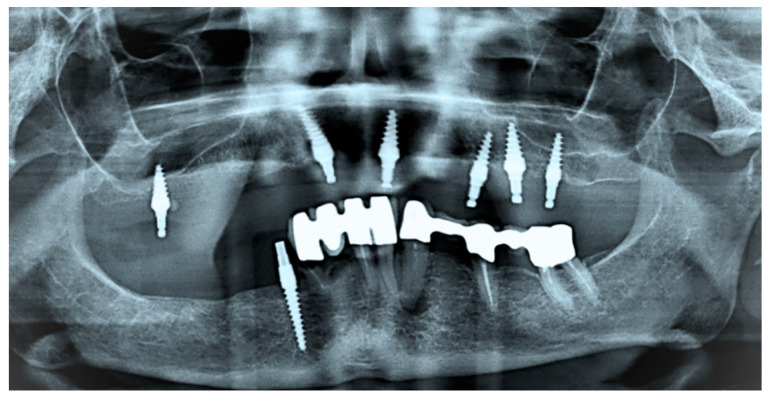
Panoramic radiograph of a geriatric patient presenting multiple single-piece implants in the maxilla with balls for prosthesis retention (implant placement alio loco several years ago). Please note that a common path of insertion has not been established for the maxillary implants. The mandibular dentition is failing, but the patient still wanted to postpone treatment.
